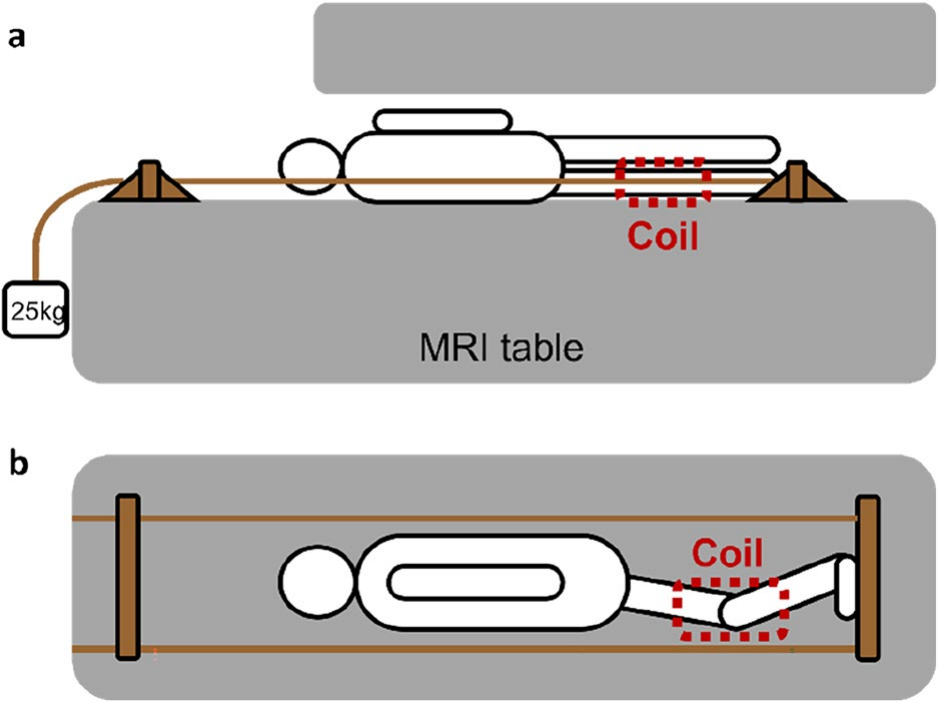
Experimental set up; (a) side view and (b) top view. The subject was positioned in lateral decubitus on the MRI table, with the foot of the dominant leg placed against a wooden plank. To load the dominant leg, a 25kg weight was attached to the plank using a cable. A coil was placed around the dominant leg for scanning purposes. The non-dominant leg was positioned to avoid any interference. A total of five scans were conducted: four scans were taken with the dominant leg loaded at angles of 90°, 60°, 30°, and 0° of knee flexion, and finally, an unloaded full lower limb scan was performed with the dominant leg at 0°.
